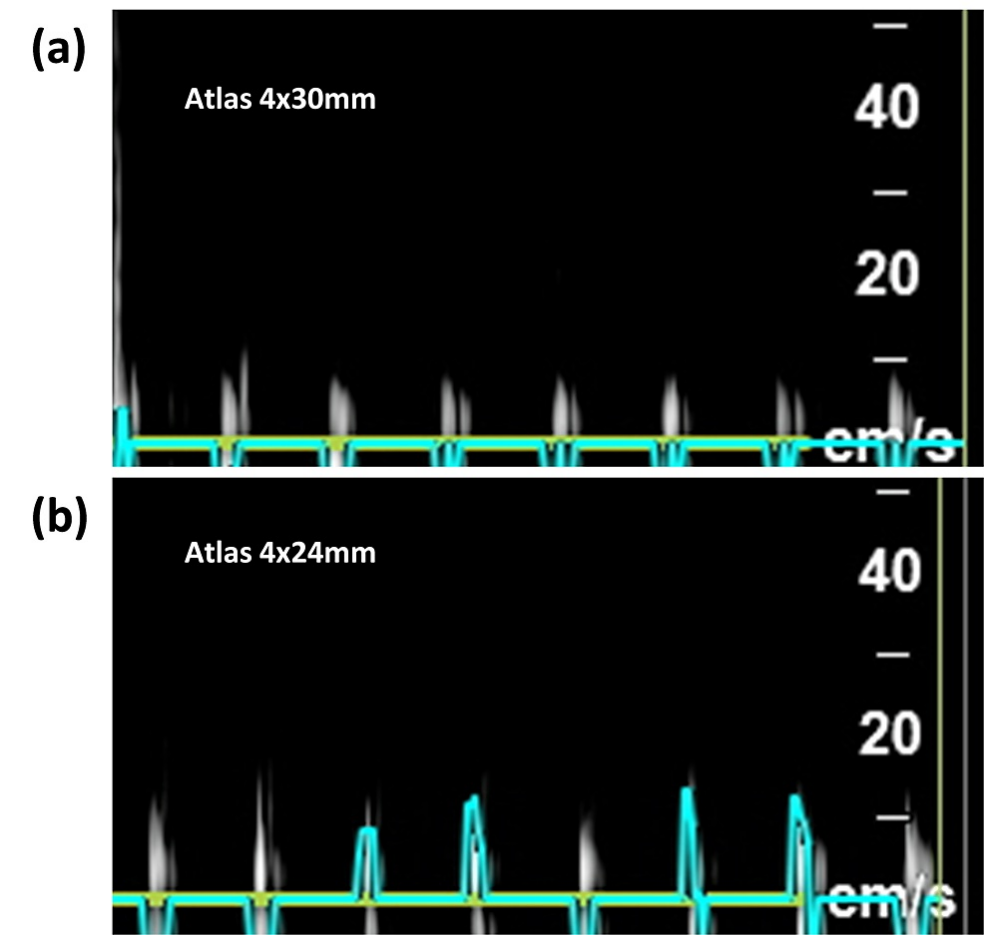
Doppler flow waveforms comparing pulsatility in Atlas stents Doppler flow characteristics in the blood vessel model after deploying the Atlas 4x30 mm stent (Figure 6a) versus the Atlas 4x24 mm stent (Figure 6b). A reduction in Vmax, PI, and EDV is seen, with a modest reduction in pulsatile flow across the stented area. Waveforms obtained utilizing the ultrasound in Doppler mode demonstrate pulsatile flow characteristics over the blood vessel model. All recordings in this figure were taken directly over the area of the stents. The Y-axis represents velocity in cm/s. mm: millimeter, cm: centimeter
